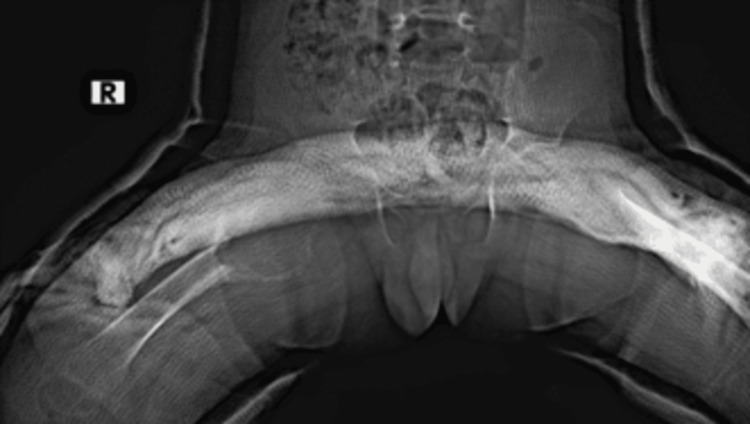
First-month control radiograph after pelvipedal cast
